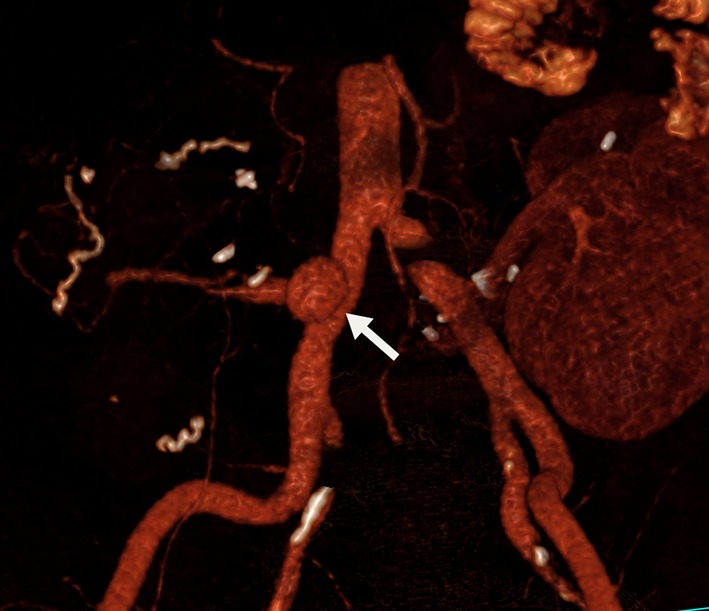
3D reconstructed CT demonstrating a 14-mm pseudoaneurysm (arrow) arising from the right common iliac artery at the origin of the Y graft arterial conduit
